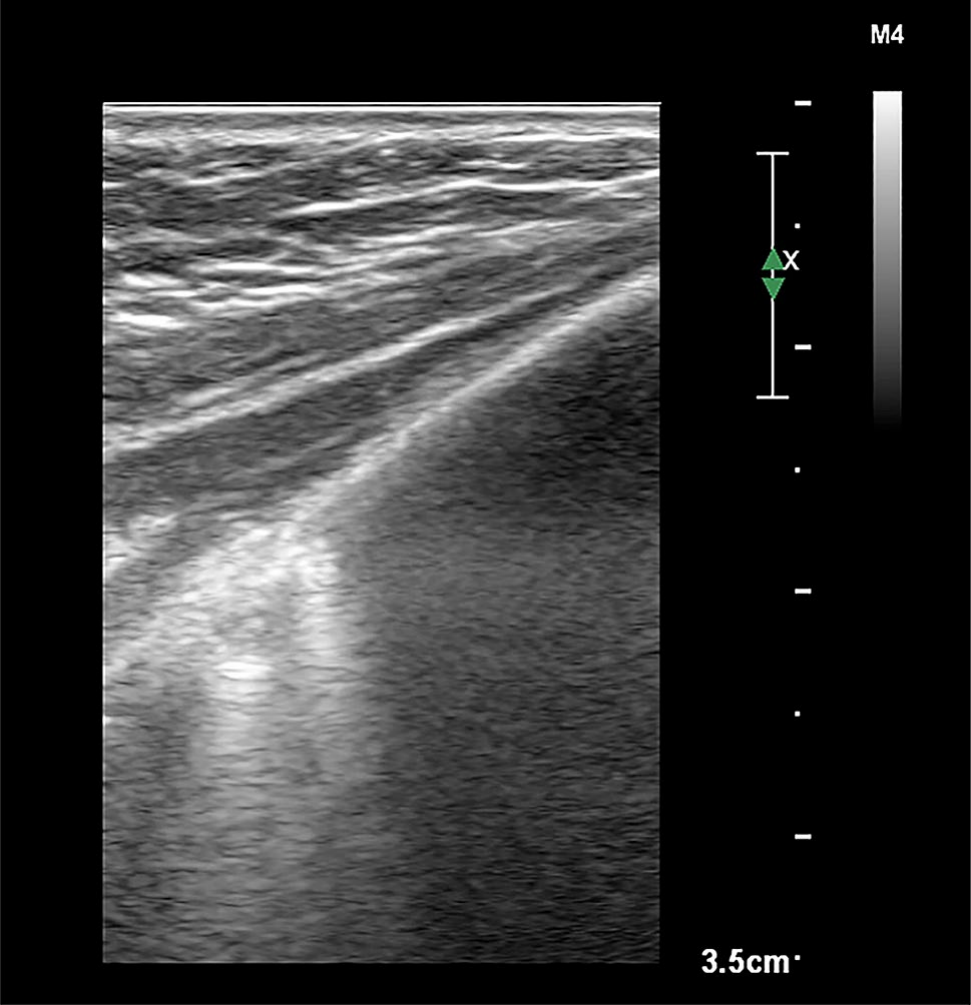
Pleural sliding and lung expansion at the lung-chest wall interface in the intercostal lung ultrasonography image. The pleurae appear as hyperechoic linear structures. Backward and forward horizontal sliding movement of the pleurae is indicated for visceral pleural movement against the parietal pleura, which is synchronized with manual ventilation. Two isolated B-lines arising from the juxta-pleural consolidation are projected vertically and thought to result from anesthesia-induced atelectasis. Statistical analyses were conducted using the SPSS software version 25.0 (IBM Inc., Armonk, NY, USA) (https://www.ibm.com/products/spss-statistics).
